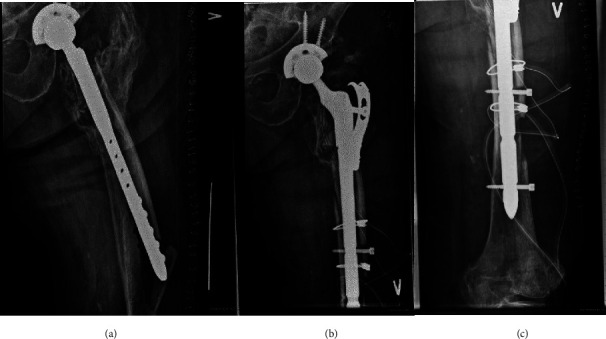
Radiographs showing (a) preoperative anterior to posterior view of the left femur in Vancouver B3 periprosthetic fracture of an uncemented femoral component; (b–c) rTHA using an Arcos cylindrical modular femoral implant with a trochanter reattachment plate and distal screw fixation.
